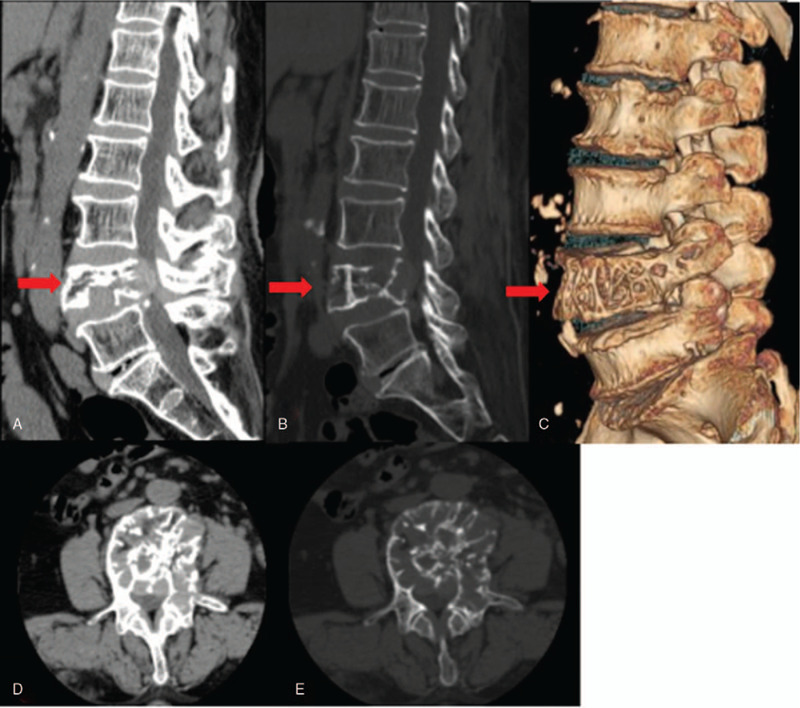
(A–E). Bone resorption and destruction changes as observed in the L4 vertebral body and left pedicle, showing a sparse bone crest structure in the L4 vertebral body and palisade-like changes. A mass-like soft tissue shadow with a size of about 2.2 × 0.8 × 2.1 cm and computed tomography value of about 102 HU were observed behind the L4 vertebral body, with a clear boundary.
